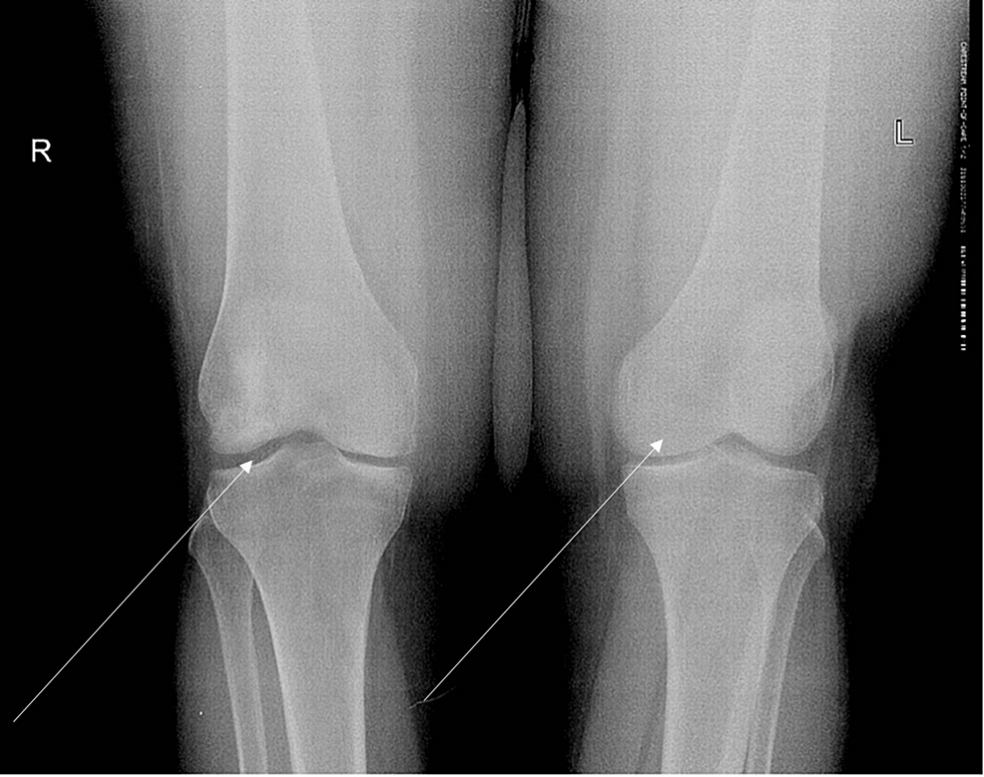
Anteroposterior radiograph of bilateral knees during the initial visit. Arrows indicate the area of possible avascular necrosis.
